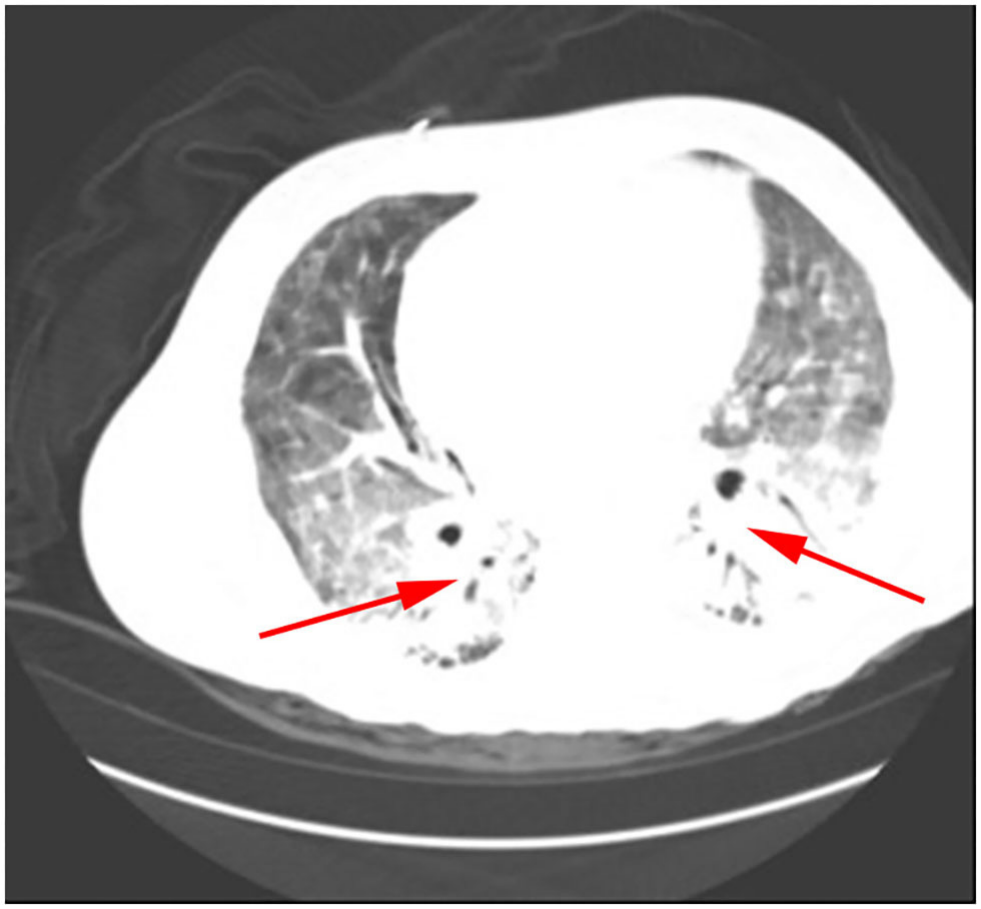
P. jirovecii* appearances on Chest CT scan in patient 3 as a large number of meshwork shadows and multiple small nodules in both lungs.
